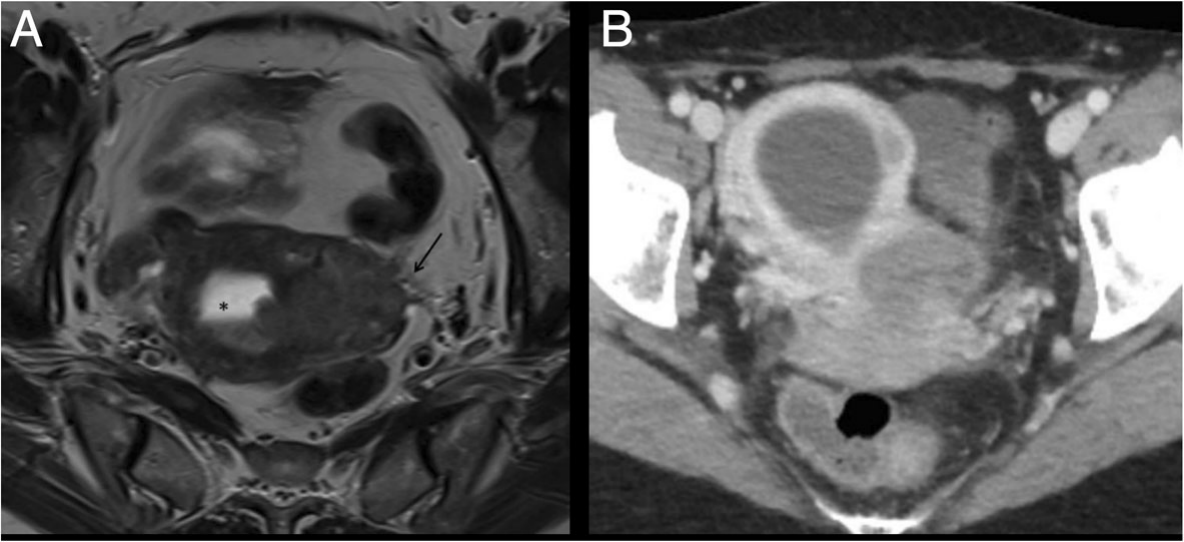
A 57-year-old woman with EC stage IIIB. Para-axial T2-w MR image (a) shows the endometrial carcinoma extending to the left parametrium (arrow). The tumour infiltrates the cervix through the internal uterine ostium leading to a retrodilation of the cavity which appears occupied by fluid (asterisk). In the same patient, the axial CT image shows the mass invading the left parametrium (b); collaterally there is a centimetric leiomyoma of the left wall of the uterus
